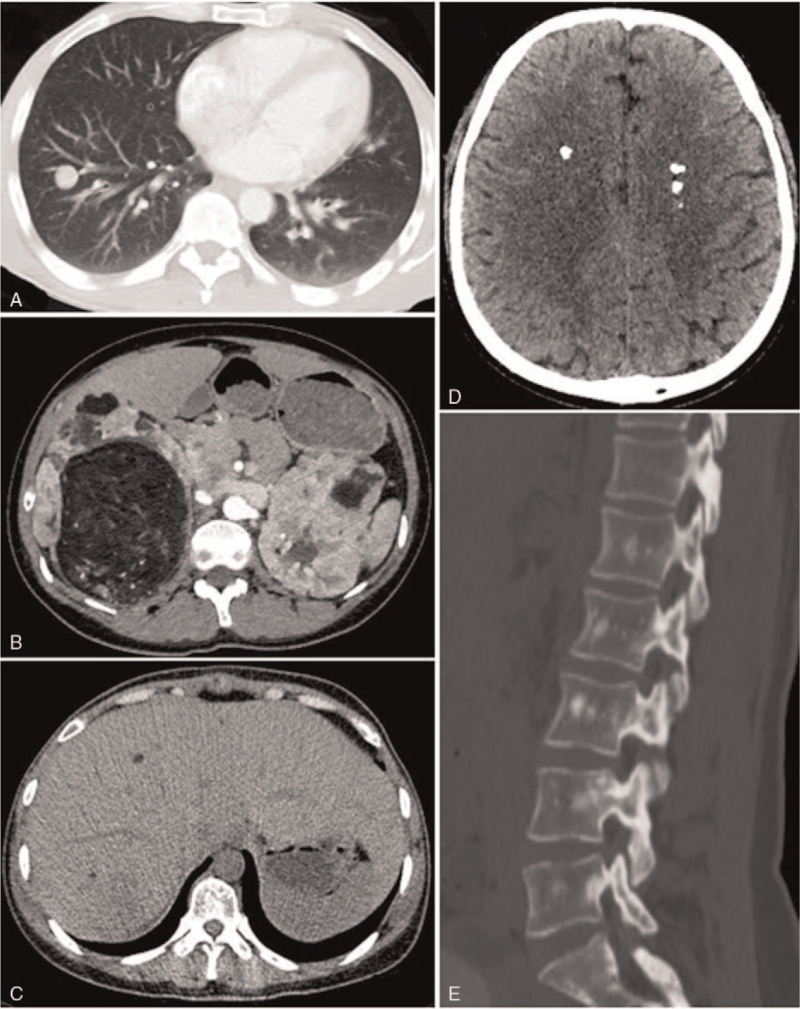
CT Imaging of the patient with TSC. (A) Lung CT scans showing presence of lymphangiomyomatosis, (B) abdomen CT scans showing angiomyolipomas, (C) liver CT showing small hamartomas, (D) brain CT showing multiple calcified subependymal nodules, and (E) spine CT showing multiple patchy sclerotic lesions (TSC = tuberous sclerosis complex and CT = computed tomography).
